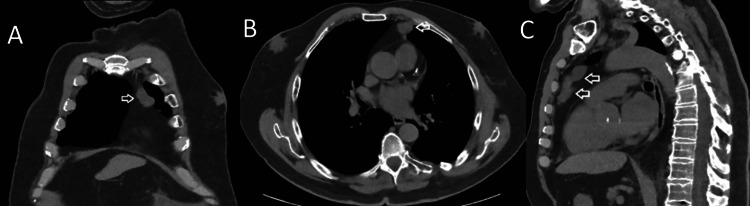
CT chest without contrast showing a new 2.2 x 1.7 x 4.5 cm soft tissue mass in the anterior mediastinum (white arrows) A: Coronal section, B: axial section, and C: sagittal section.
